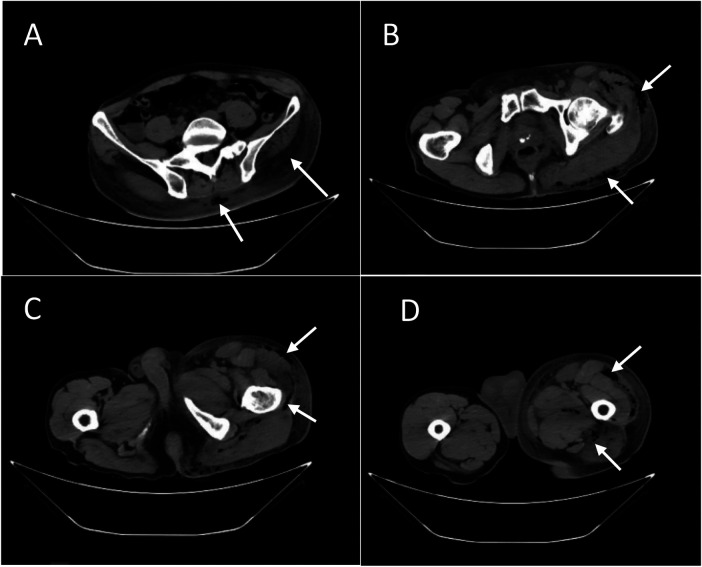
(A–D) the CT images obtained at the time of the patient's hospital admission.
